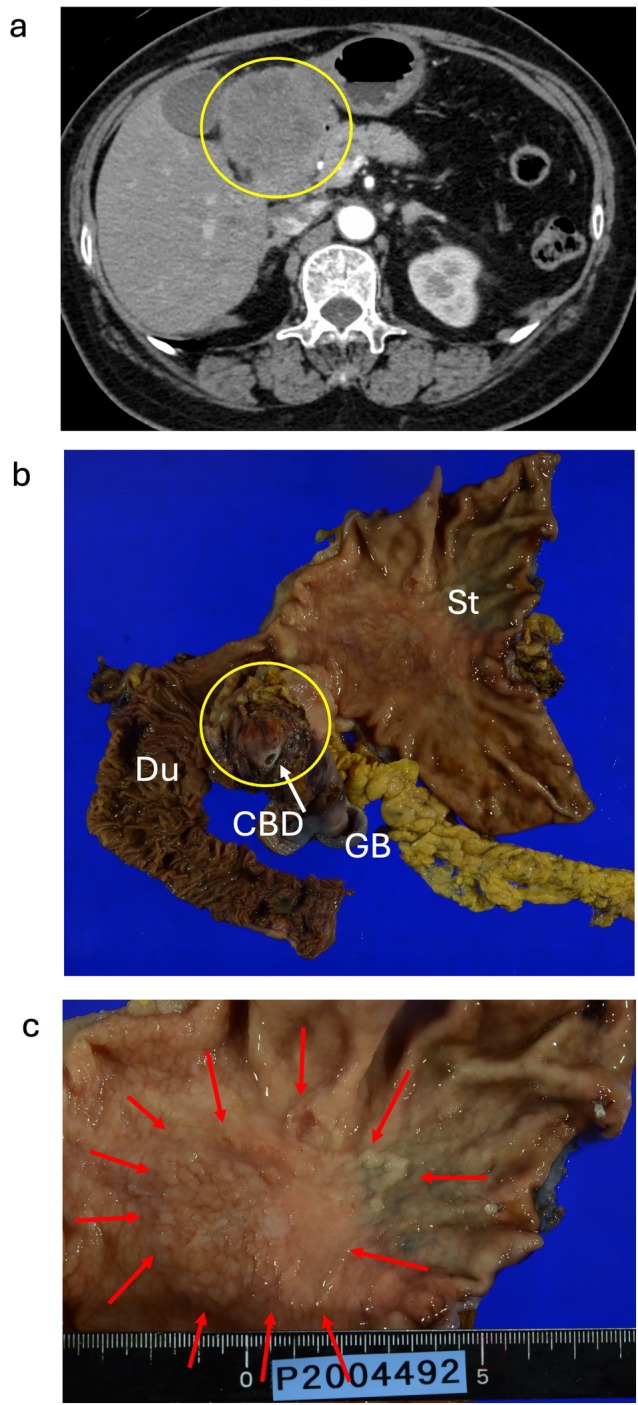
Cancer of unknown primary (CUP) and early gastric cancer. (a) A computed tomographic image of CUP shows a heterogeneous density mass at the portal hepatis, growing to the gall bladder and the pancreatic head. Yellow circle indicates the main tumor. (b) Surgically resected CUP tissue. The main tumor involves to the duodenum (Du), gall bladder (GB), and common bile duct (CBD). The tumor is separated from the stomach (St). Yellow circle indicates the main tumor. (c) Early gastric cancer forms a laterally spreading tumor. Red arrows indicate the lateral margin.
